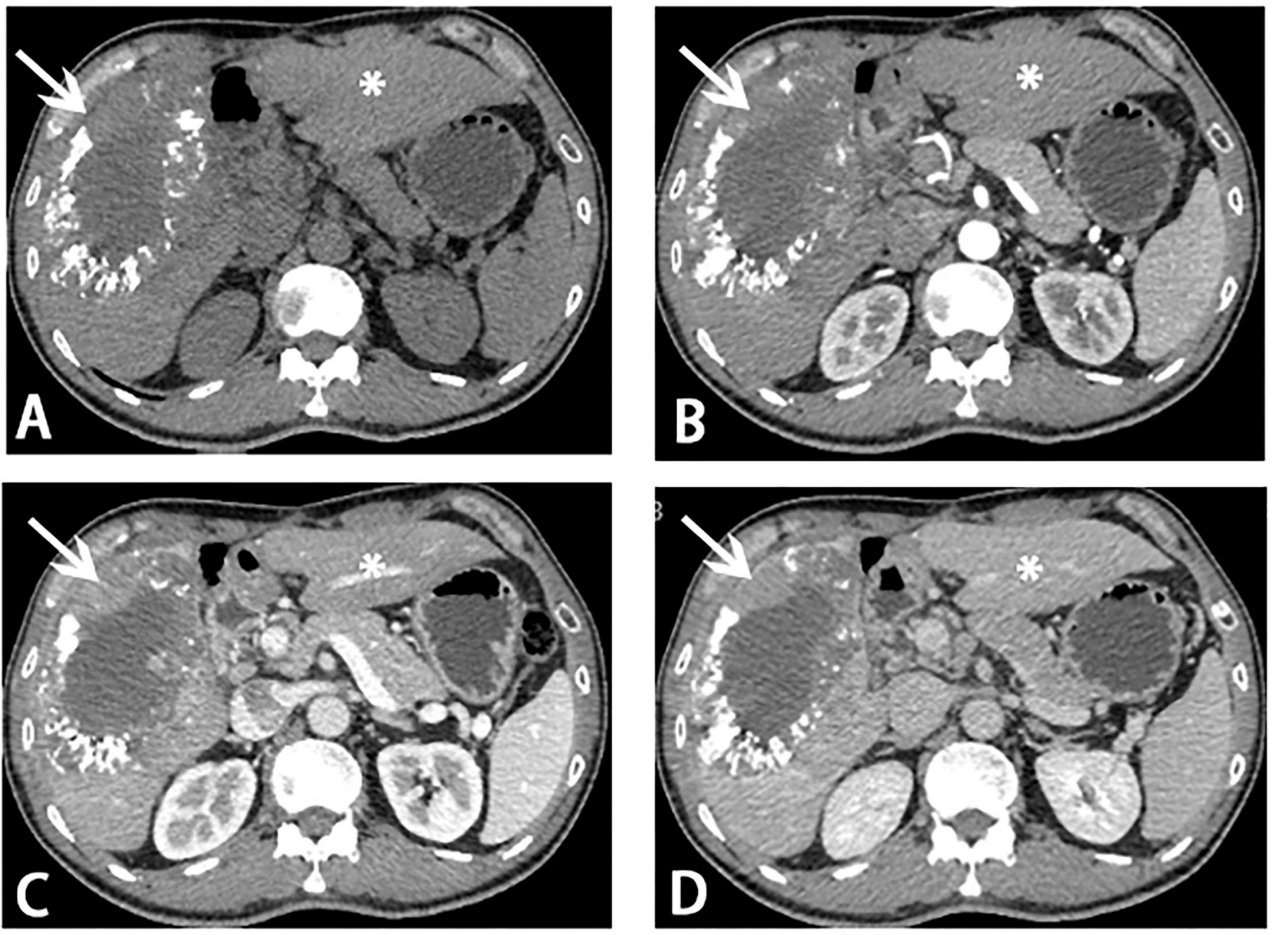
Computed tomography (CT) scans before stage II ALPPS. Reduce of tumor load (white arrow) and hypertrophy of segments 2 and 3 (asterisk) was showed on CT scans. (A) Plain scan; (B) arterial phase; (C) portal venous phase; (D) venous phase.
